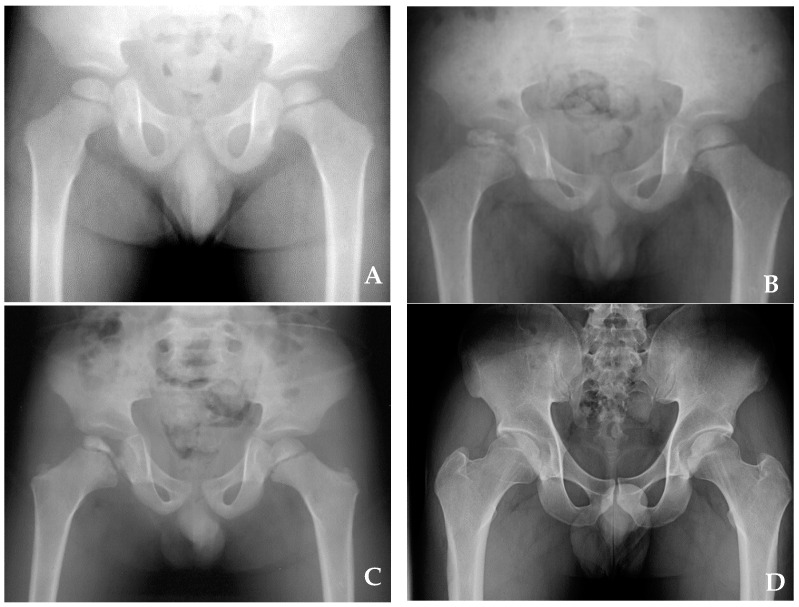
Anteroposterior radiograph of patients at the age of 2 years. Initial (A), fragmentation (B), reossification (C), and residual (D) stages.
